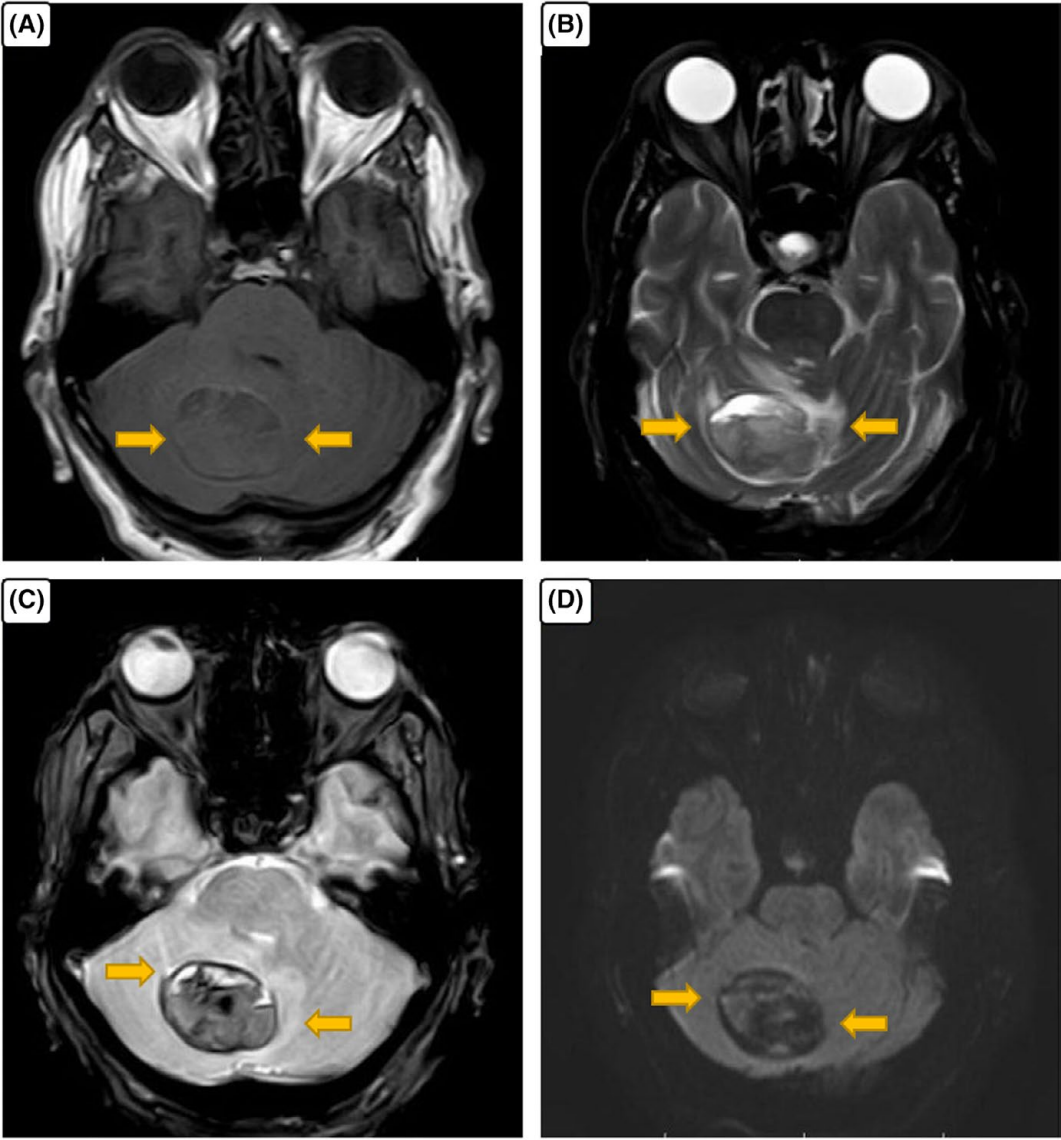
A, B, A right cerebellar mass contain fluid‐fluid level with heterogeneous low to intermediate signal intensity in T1W and intermediate to high signal intensity in T2W; C, Dark signal intensity in T2*sequence with blooming effect representing acute hemorrhage; D, No diffusion restriction is present in DWI sequence
